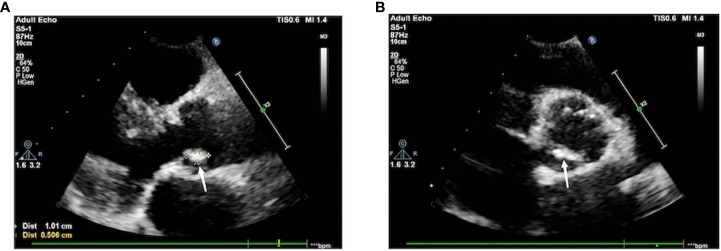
Cardiac color Doppler ultrasound. There is a slightly echogenic mass measuring approximately 10 mm × 5mm on the aortic valve, with low mobility. (A) The long-axis view of the left ventricle next to the sternum shows an attached vegetation on the aortic valve without coronary valve involvement. (B) The short-axis view of the left ventricle next to the sternum shows the formation of a vegetation on the aortic valve without coronary valve involvement. A small amount of fluid-filled dark area is visible in the pericardial cavity, with an anterior–posterior diameter of 3 mm.
